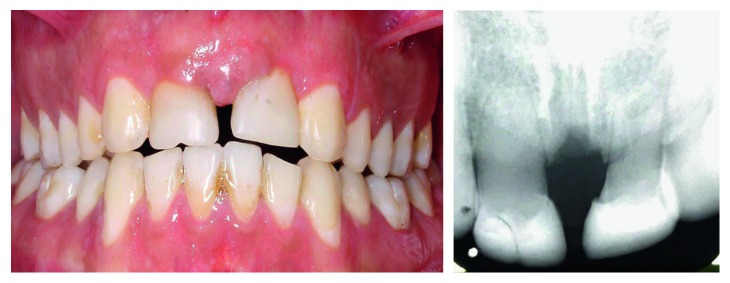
Radiographic and clinical view at the 13-year follow-up.
